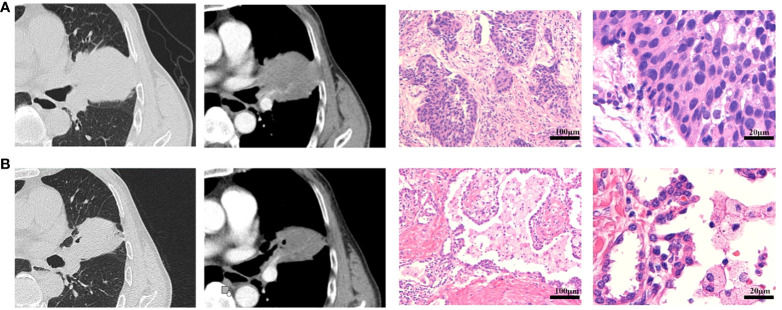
Radiological and pathological response of neoadjuvant immunotherapy plus chemotherapy. (A) The CT imaging and pathological diagnosis at baseline. (B) The CT imaging after two doses of neoadjuvant immunotherapy plus chemotherapy, and the pathological results after surgery. This was a 70-year-old male patient with smoking history, who was diagnosed as cT3N1M0 (stage IIIA) squamous cell carcinoma at baseline. After two doses of neoadjuvant immunotherapy plus chemotherapy, the patient achieved SD according to RECIST v1.1 with CT imaging assessment of 24% shrinkage of tumor. This patient underwent R0 resection with sleeve lobectomy and the pathological results with pCR. The regression bed is characterized by dense immune infiltrates with features of activation (tertiary lymphoid structure and dense tumor infiltrating lymphocytes infiltrates), along with features of cell death.
